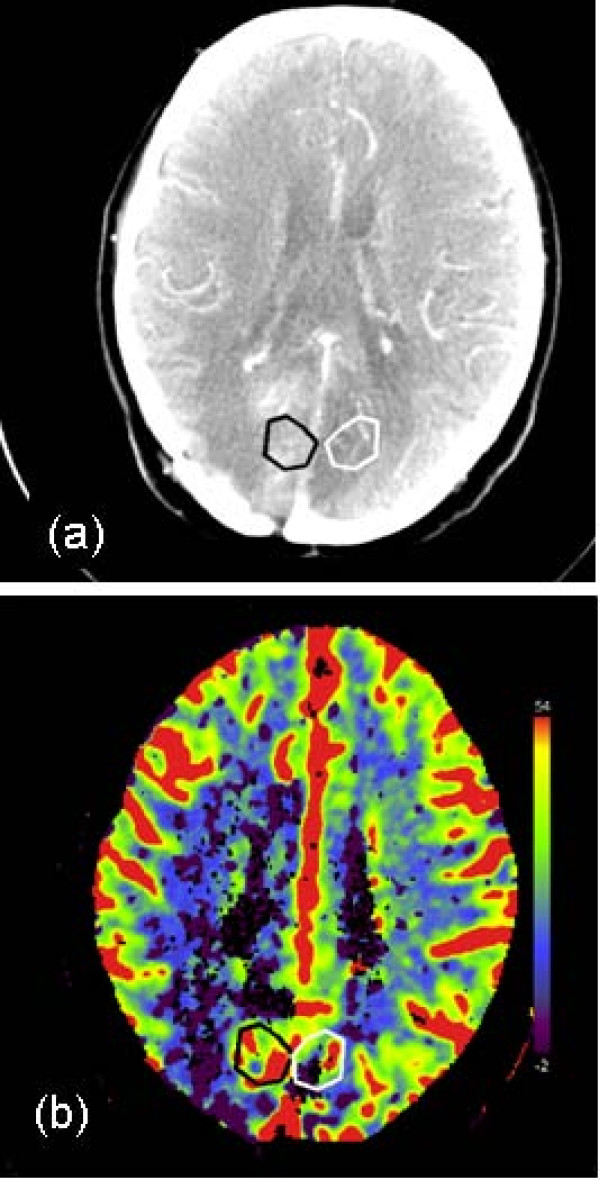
Transverse CT (Computer Tomography) image (a) and CBV (Cerebral Blood Volume) map (b) in a patient affected by a recurrence of glioblastoma. In both the images, the hand-drawn ROI (region of interest) corresponding to the tumor and the contralateral ROI are displayed in black and white, respectively.
